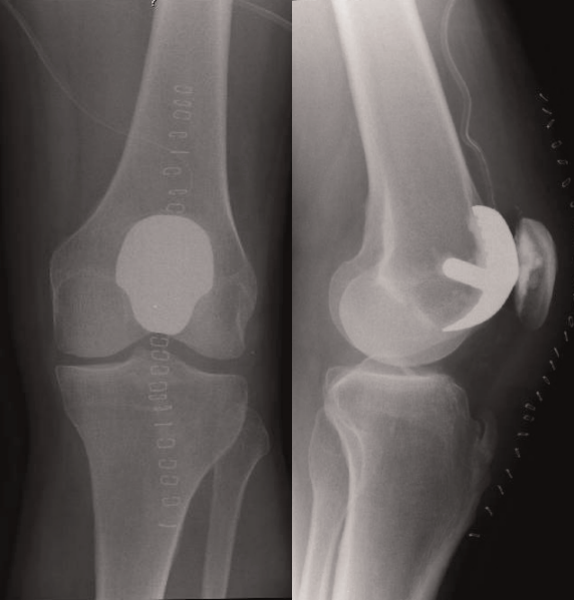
Radiograph of left knee after conversion to the Richards type II patellofemoral arthroplasty.
